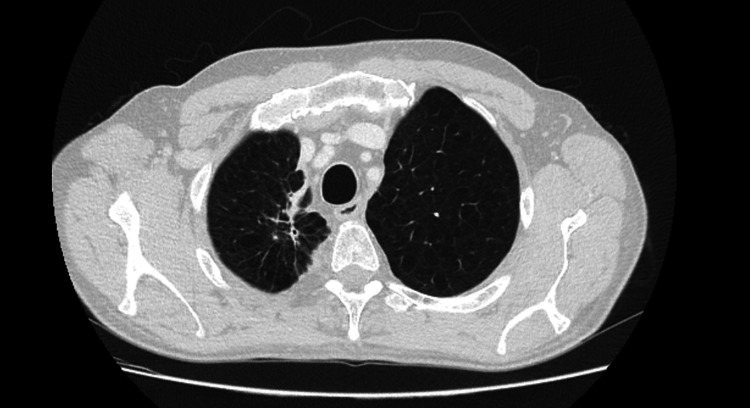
Follow-up abdominal and chest CT after finishing pembrolizumab and receiving SBRT performed in December 2022
